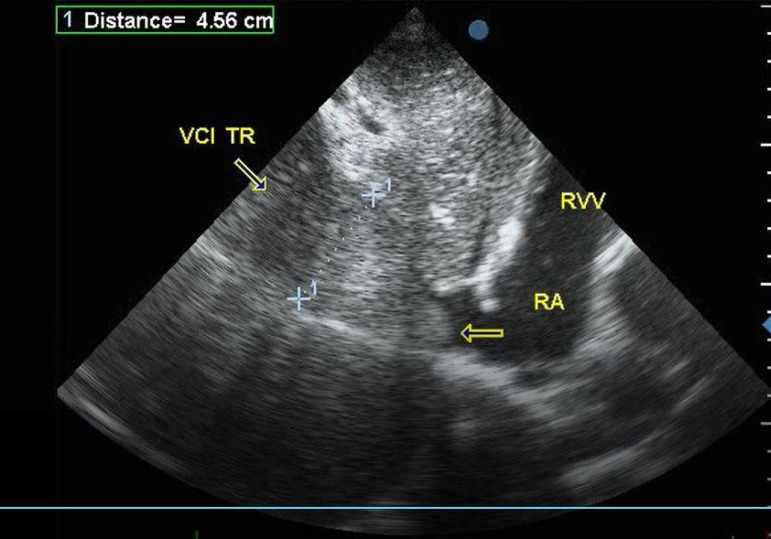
Echocardiogram in female patient with IVC tumor and supradiaphragmatic
